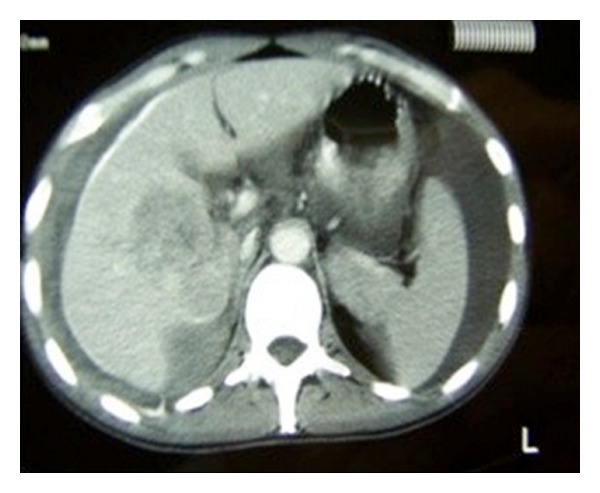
CT abdomen showing the liver mass and hemoperitoneum.
